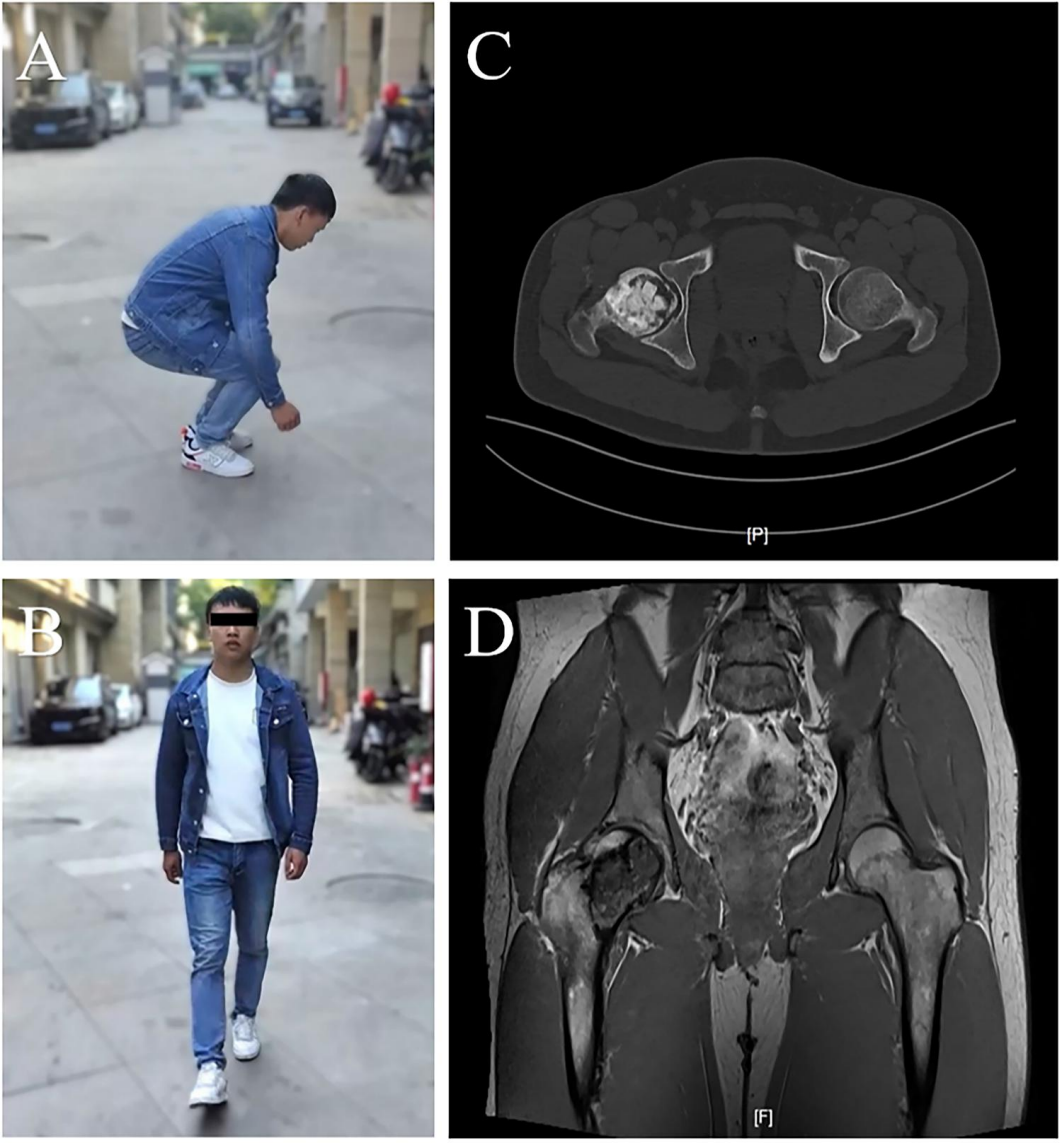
Functional recovery and radiographic follow-up at 9 months postoperatively. (A) Squatting activity without obvious limitation. (B) Walking activity without apparent restriction. (C,D) Follow-up imaging demonstrating no obvious resorption in the bone grafting area.
